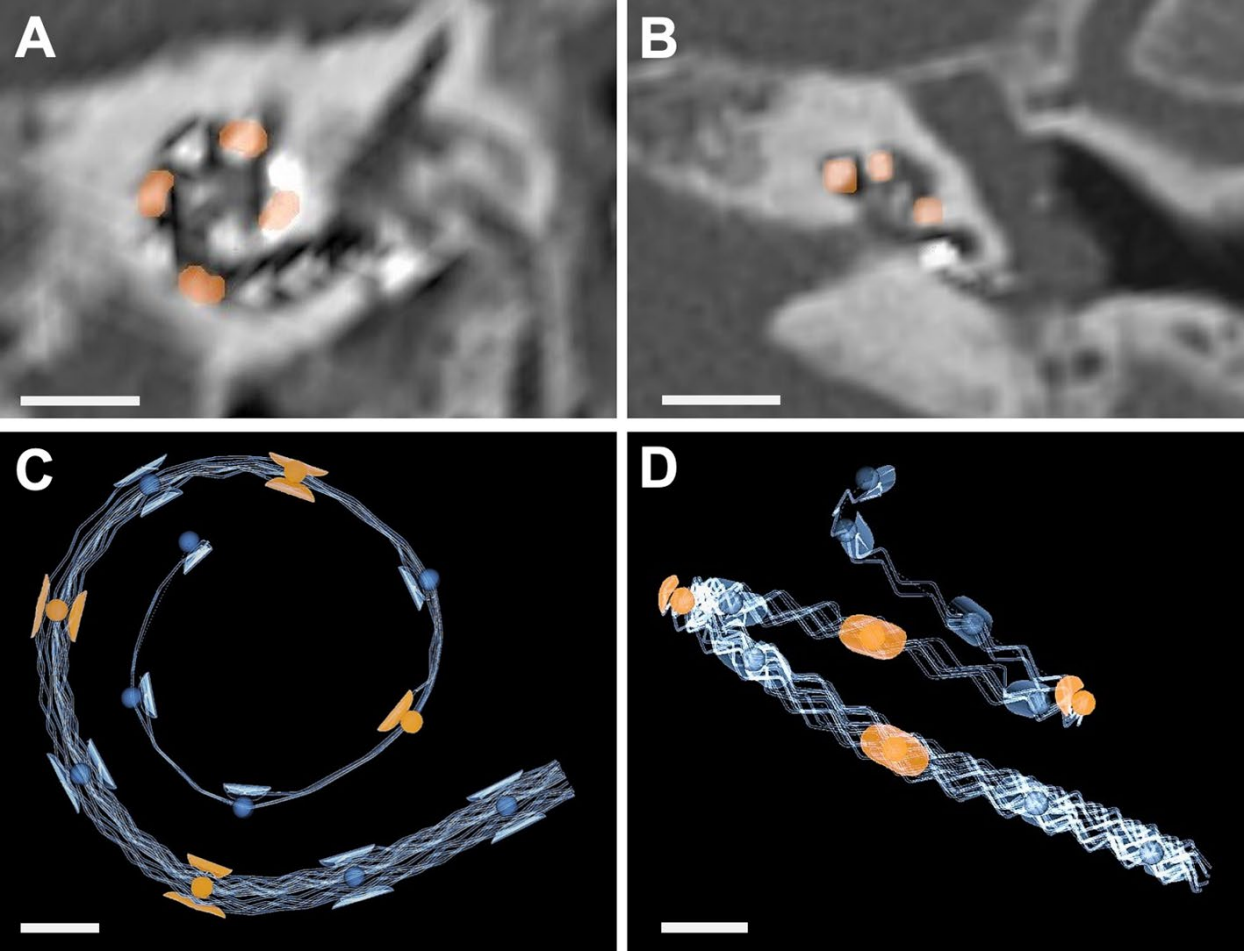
Postoperative angular insertion depth determination. A Postsurgical CT-scan oblique coronal view. Electrode array positioned along the lateral cochlear wall. Electrode contacts entering the cochlea 90°, 180°, 270° and 360° are marked in orange. B Postsurgical CT-scan axial view. Scale bar 5 mm. C Three-dimensional electrode array reconstruction from oblique coronal view. Electrode contacts entering the cochlea 90°, 180°, 270° and 360° are marked in orange. D Three-dimensional electrode array reconstruction from axial view. Scale bar 1 mm
